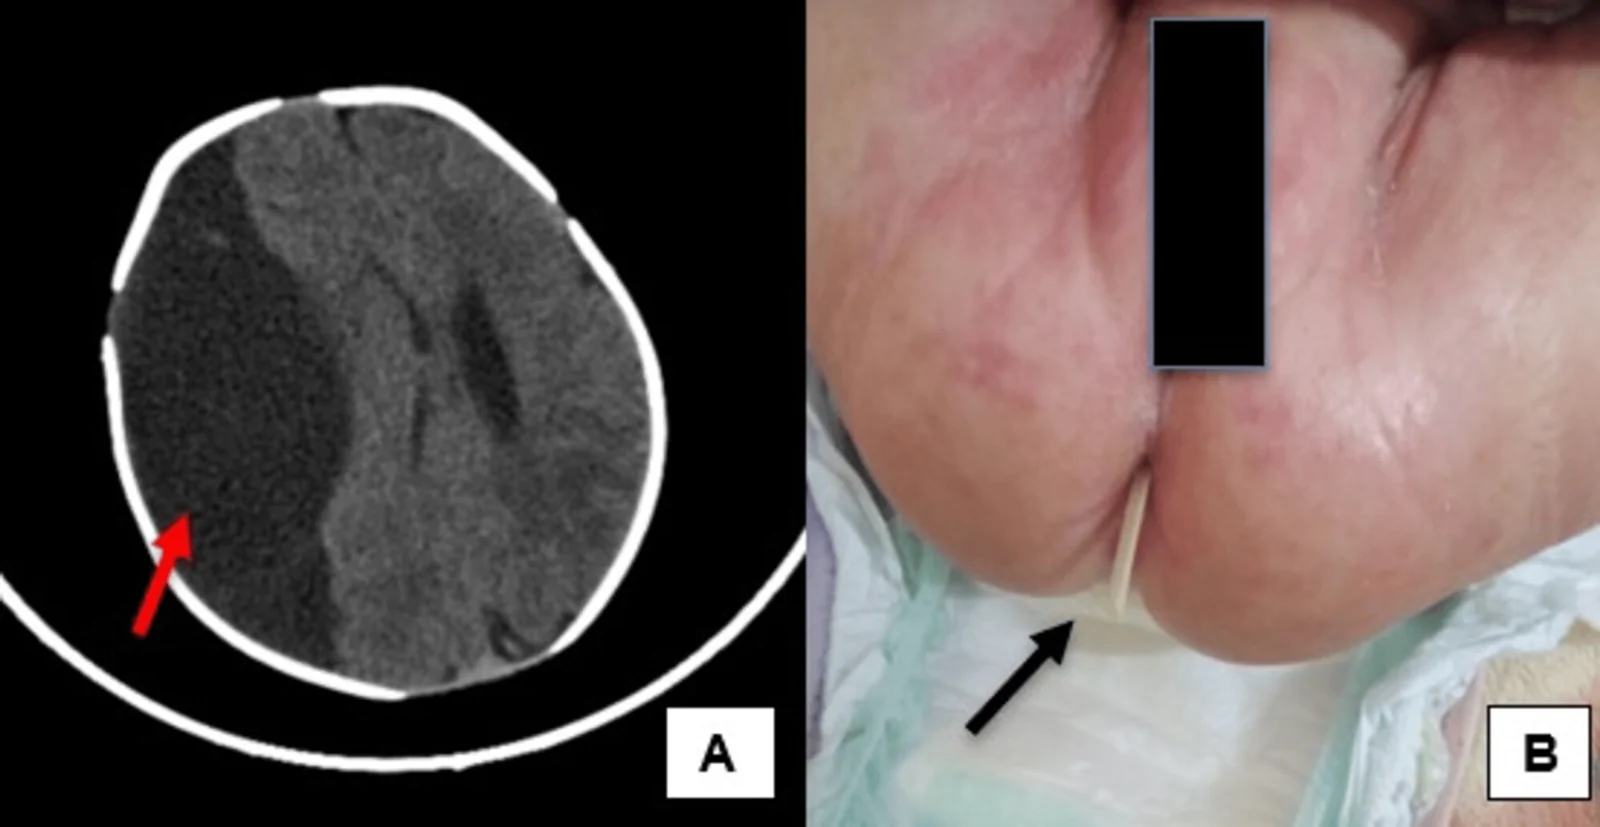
(A) Preoperative brain CT scan obtained at one month of age, demonstrating a right frontoparietal arachnoid cyst (red arrow) causing significant mass effect, with midline shift and compression of the right lateral ventricle. (B) Clinical photograph showing transanal extrusion of the distal end of the cystoperitoneal shunt catheter (black arrow).
